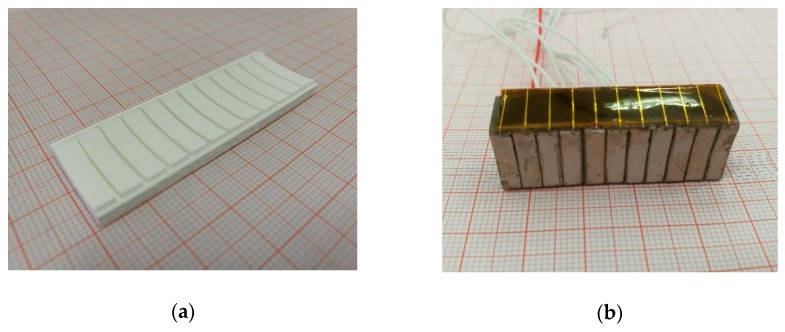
The photos of the 3D-printed (a) ceramic and (b) ultrasonic arrays.
